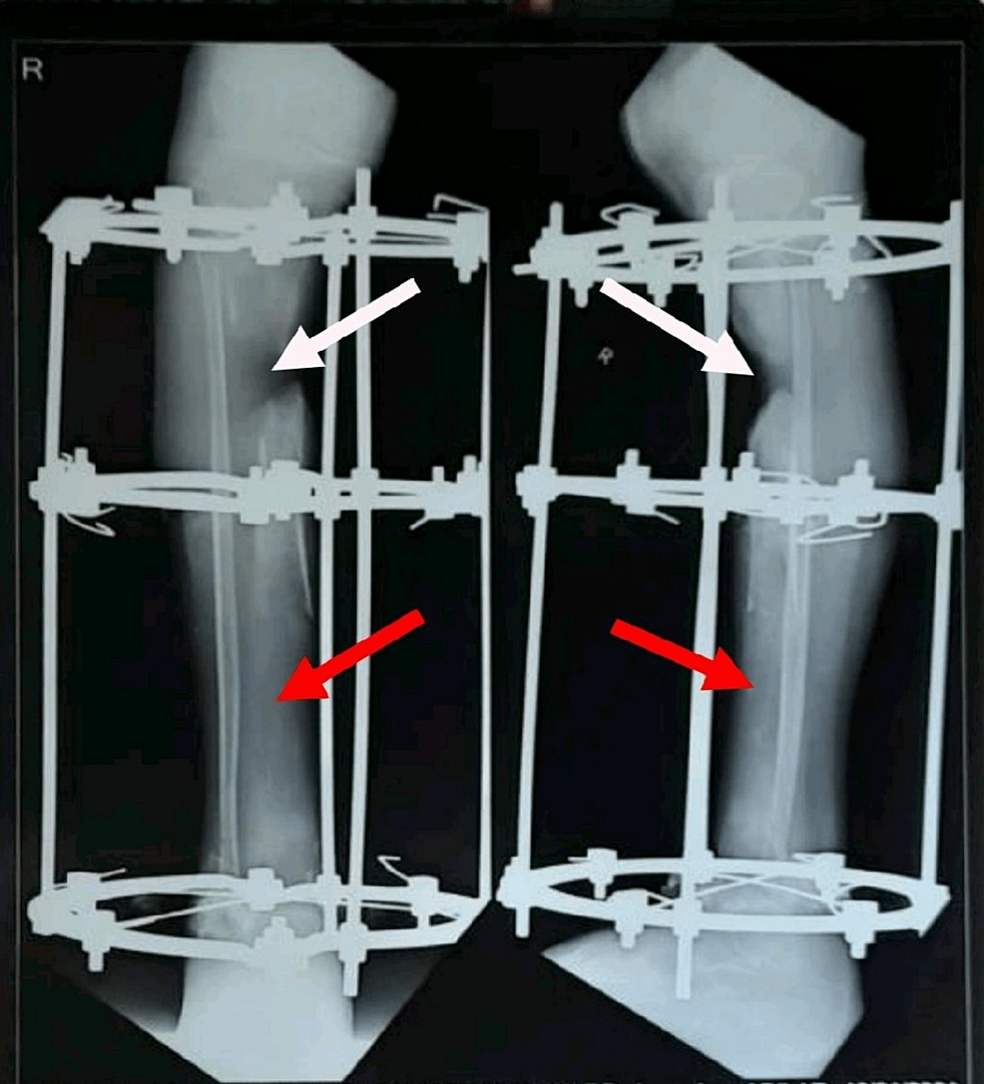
X-ray of the right lower leg (AP and lateral view) with Ilizarov ring in situ showing bone loss of approximately 4cm over the proximal 3rd tibia (white arrows) and new bone formation over the distal 3rd tibia (red arrows) anteroposterior: AP
